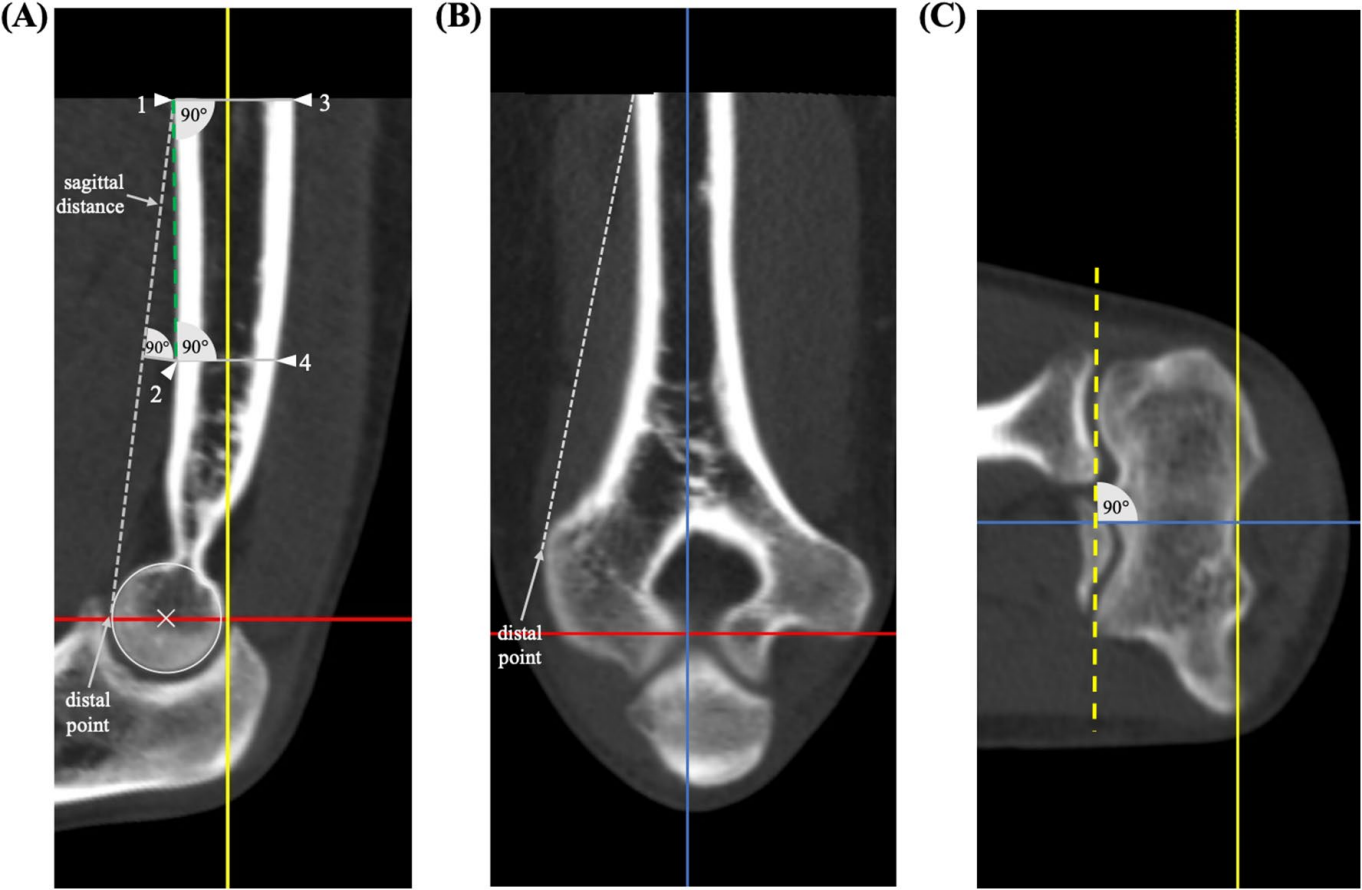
CT standard planes and determination of humeral shaft axis. The determination of the humerus shaft axis as applied for defining the standard planes is described in the section ‘humerus shaft axis’ and is depicted in this figure (A). The determination of the coronal humerus shaft axis (B) is achieved via the same approach as described for the sagittal view. The ‘distal point’ is defined as the most volar location on the trochlea in the sagittal view and the most lateral location on the lateral epicondyle in the coronal view and serves for determination of the sagittal distance. Red line: axial plane, bisects the trochlea in the sagittal view. Yellow line: coronal plane, humerus shaft axis in the sagittal view and parallel to the joint surface (dashed yellow line) in the axial view (C). Blue line: sagittal plane, humerus shaft axis in the coronal view. Grey dashed line: sagittal distance. Green dashed line: connection of point 1 and 2. Circle: trochlea surface (color figure online)
